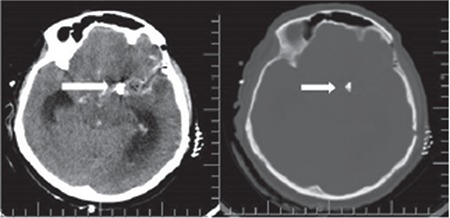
The patients preoperative axial brain computed tomography showing aneurysm clips
